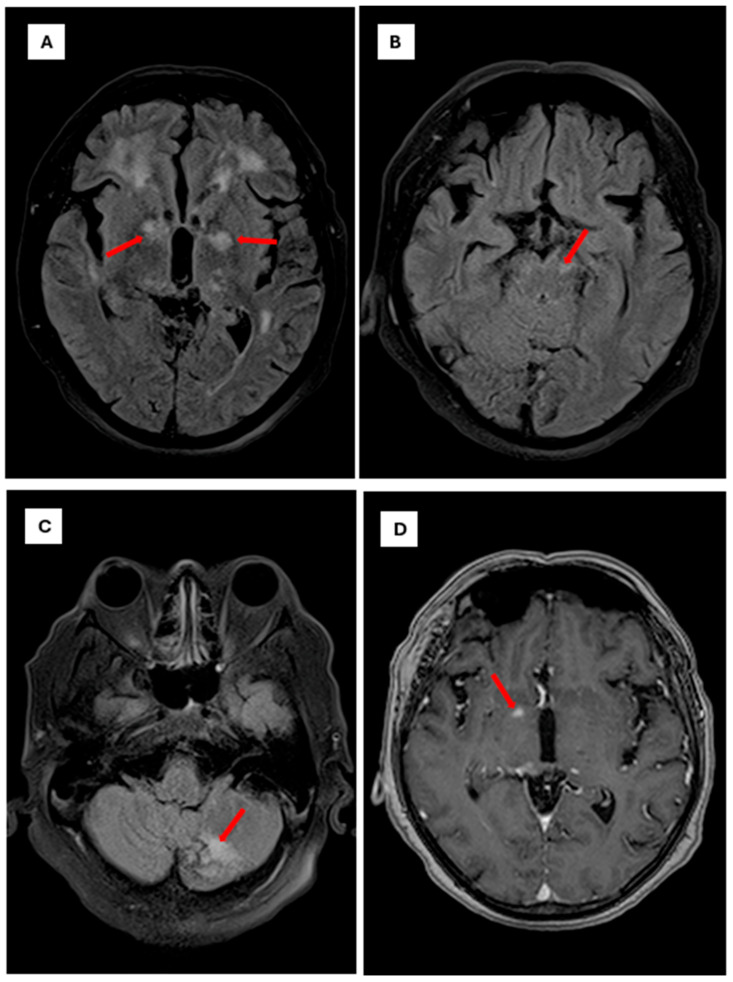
Brain magnetic resonance imaging findings in Cryptococci meningoencephalitis. Note: Axial brain MRI sequences demonstrating characteristic findings of cryptococcal meningoencephalitis The scale bar is set to 10 mm. (A): Axial FLAIR sequence showing focal hyperintense lesions involving the white matter and bilateral gangliobasal region, particularly the globus pallidus (red arrows). (B): Axial FLAIR sequence showing a focal hyperintense lesion affecting the left mesencephalic peduncle (red arrows). (C): Axial FLAIR sequence showing a focal hyperintense lesion involving the posterior lobe of the left cerebellar hemisphere (red arrow). (D): Axial T1-weighted sequence with gadolinium contrast showing a pinpoint focal enhancement in the right globus pallidus (red arrow).
